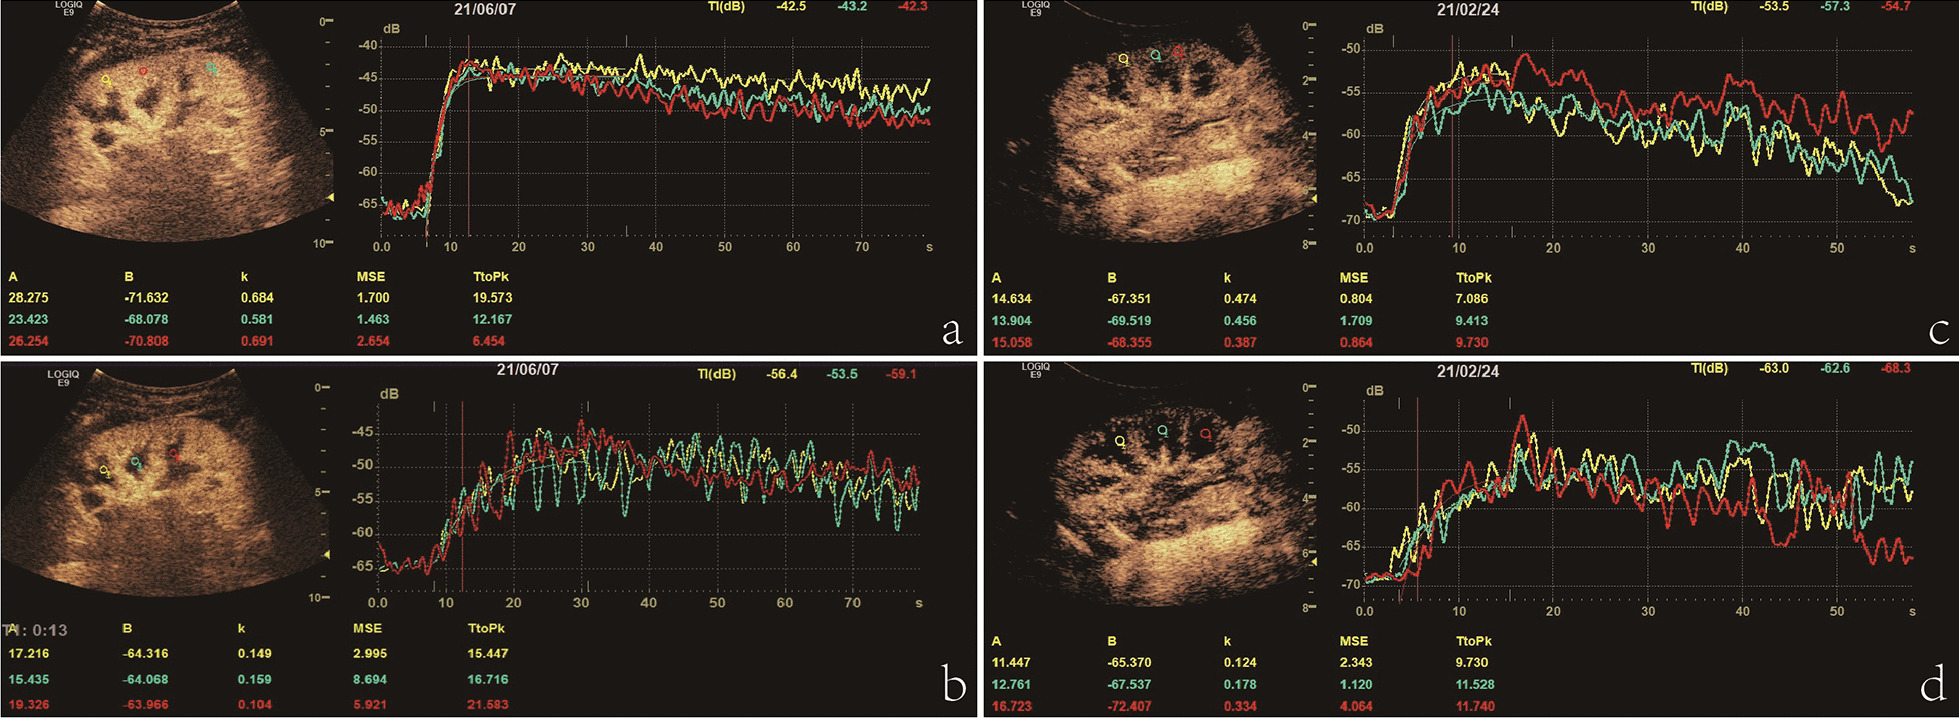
Quantitative analysis of contrast-enhanced ultrasonography (CEUS) images based on time-intensity curve fittings in a child without kidney disease (a & b) and in a pediatric patient with CKD stage 5 (c & d). Three regions of interest (ROIs) were placed in the cortices, and three ROIs were placed in the medullas. Quantitative parameters of wash-in blood flow, including A, B, k, and TtoPk, were generated automatically for each ROI
